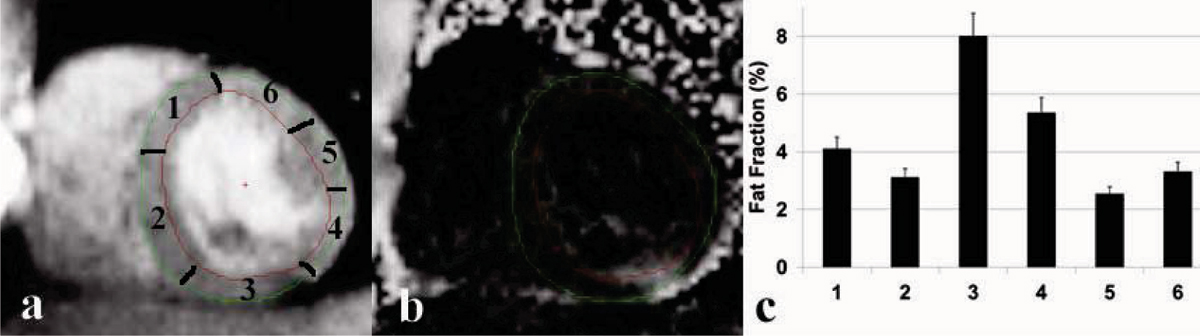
The fat fraction comparisons shown on the MRI is greater than those of MRS, likey due to noise bias contributing to the fat fraction calculation.
